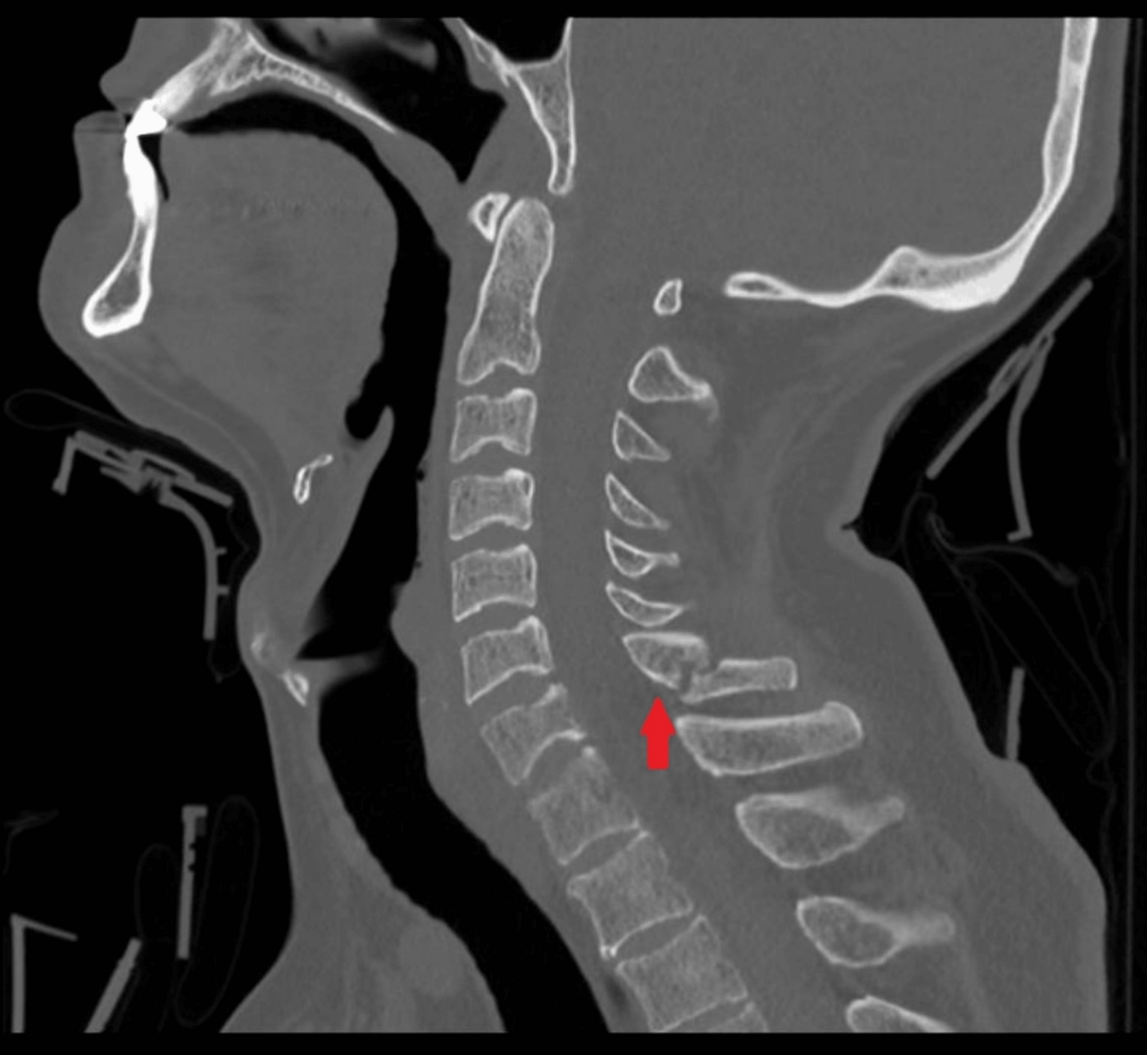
Sagittal CT image of the cervical spine demonstrating an oblique fracture (red arrow) through the mid to proximal portion of the C7 spinous process. The fracture line is clearly visualized extending obliquely through the spinous process, with minimal displacement and preserved alignment of the adjacent vertebral bodies. CT: computed tomography
